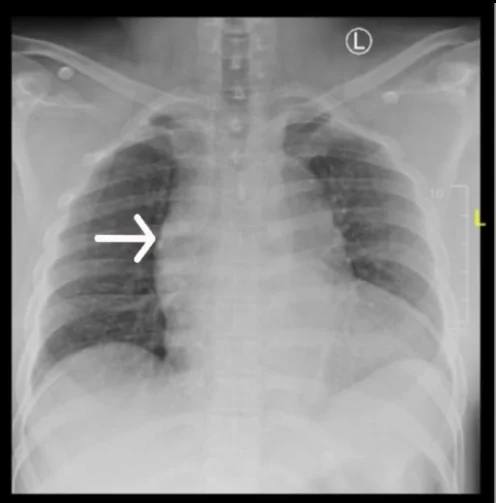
Chest radiograph demonstrating a widened mediastinum, a classic finding in aortic pathology.
